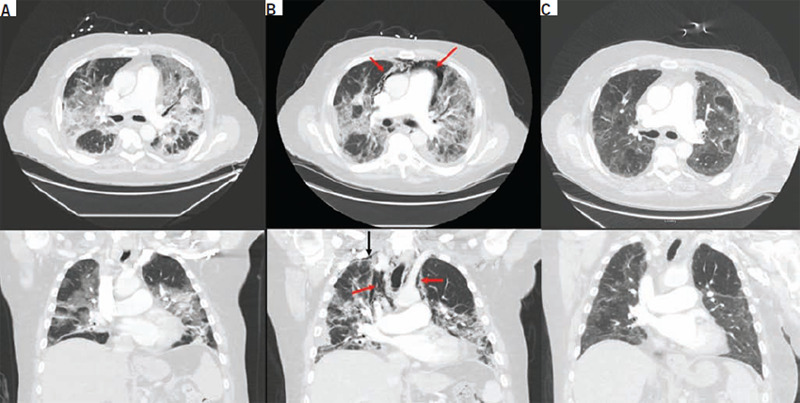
The patient's chest CT (top row, axial views; bottom row, coronal views) on hospital day 11 (A), revealing bilateral infiltrates consistent with COVID-19 pneumonia. Chest CT on hospital day 21 (B), showing pneumomediastinum (red arrows) and a small apical right pneumothorax (black arrow) and bilateral infiltrates. At 12-week follow-up (C), the bilateral infiltrates and pneumomediastinum have resolved.
